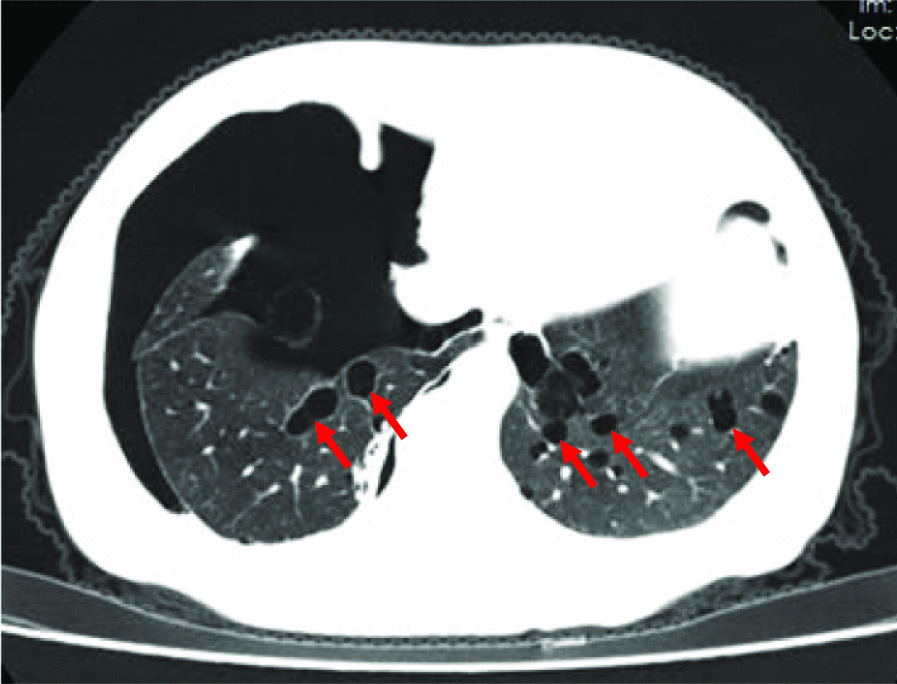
BHD syndrome in a 54-year-old female. Chest CT image shows multiple cysts of varying sizes and irregular shapes predominantly in the lower lungs, and pneumothorax on the right side
